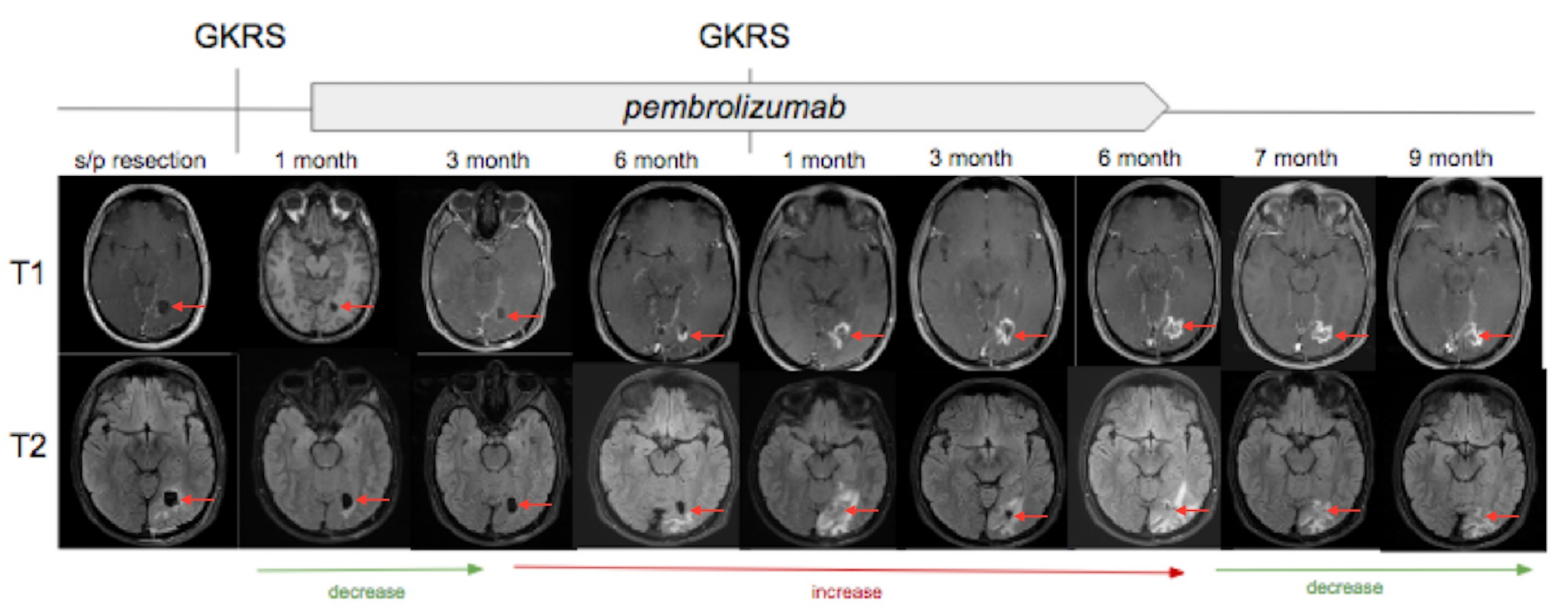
T1-weighted with gadolinium (top) and T2 fluid attenuated inversion recovery images (bottom) associated with the left occipital lobe lesion that underwent postsurgical treatment with Gamma Knife radiosurgery (GKRS) followed by initiation of pembrolizumab one week and two days later. From left to right, magnetic resonance images obtained at one month, three months, and six months status post first GKRS treatment. Repeat-GKRS was then performed while concurrent treatment with pembrolizumab. From left to right, magnetic resonance images obtained at one month, three months, six months, seven months, and nine months status post repeat-GKRS. Interval increase and decrease in T2 signal and surrounding enhancement are denoted with arrows at bottom of images.
